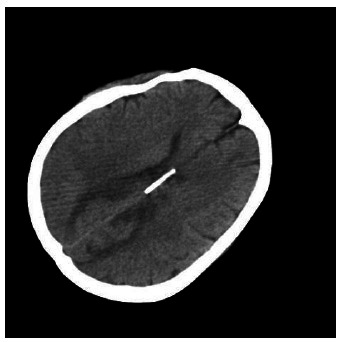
CT brain imaging on day 5 after onset of illness. CT shows striated hyperdense shadows in the region of the pericallosal segment of the anterior cerebral artery, along with newly observed hypodense areas with poorly defined borders in the parietal lobe proximal to the midline bilaterally and in the area of corpus callosum compression.
